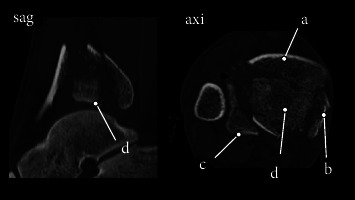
CT at the time of the injury. (a) Anterior fragments. (b) Medial fragments. (c) Posterolateral fragments. (d) Die-punch fragments.
